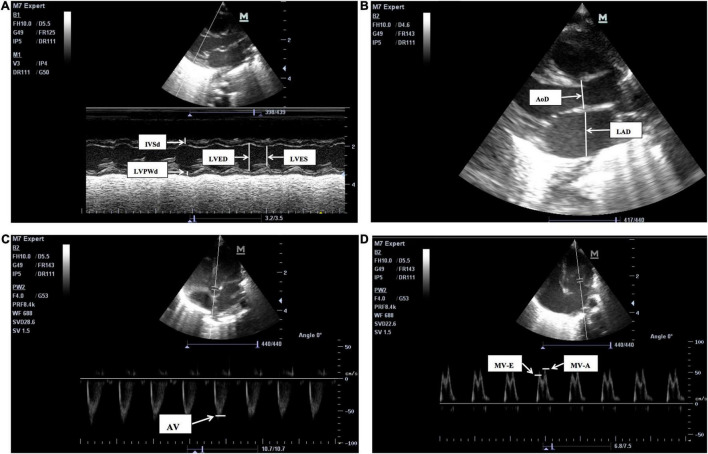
The two-dimensional (2D), M-mode or Doppler echocardiographic measurements pictures for LV structure and function. (A) The M-mode echocardiographic measurements for left ventricle and interventricular septum. IVSd, end-diastolic interventricular septum, LVPWd, left ventricular posterior wall end-diastolic thickness, LVED, Left ventricular end-diastolic, LVES, left ventricular end-systolic, LVFS, left ventricular fractional shortening, LVEF, left ventricular ejection fraction. LVFS (%), (LVED-LVES)/LVED × 100, LVEF is calculated by Teichholtz method. (B) The 2D measurements of left atrium diameters (LAD) and aortic root diameters (AoD), were taken in end-systole at the level of the aortic valves. LA/AO = LAD/AoD. (C) The pulsed wave Doppler measurements for aortic valve flow rate (AV). (D) The pulsed wave Doppler measurements for peak mitral valve flow rate E(MV-E), peak mitral valve flow rate A(MV-A), the peak mitral valve flow rate E to A (MV-E/A), MV-E/MV-A.
